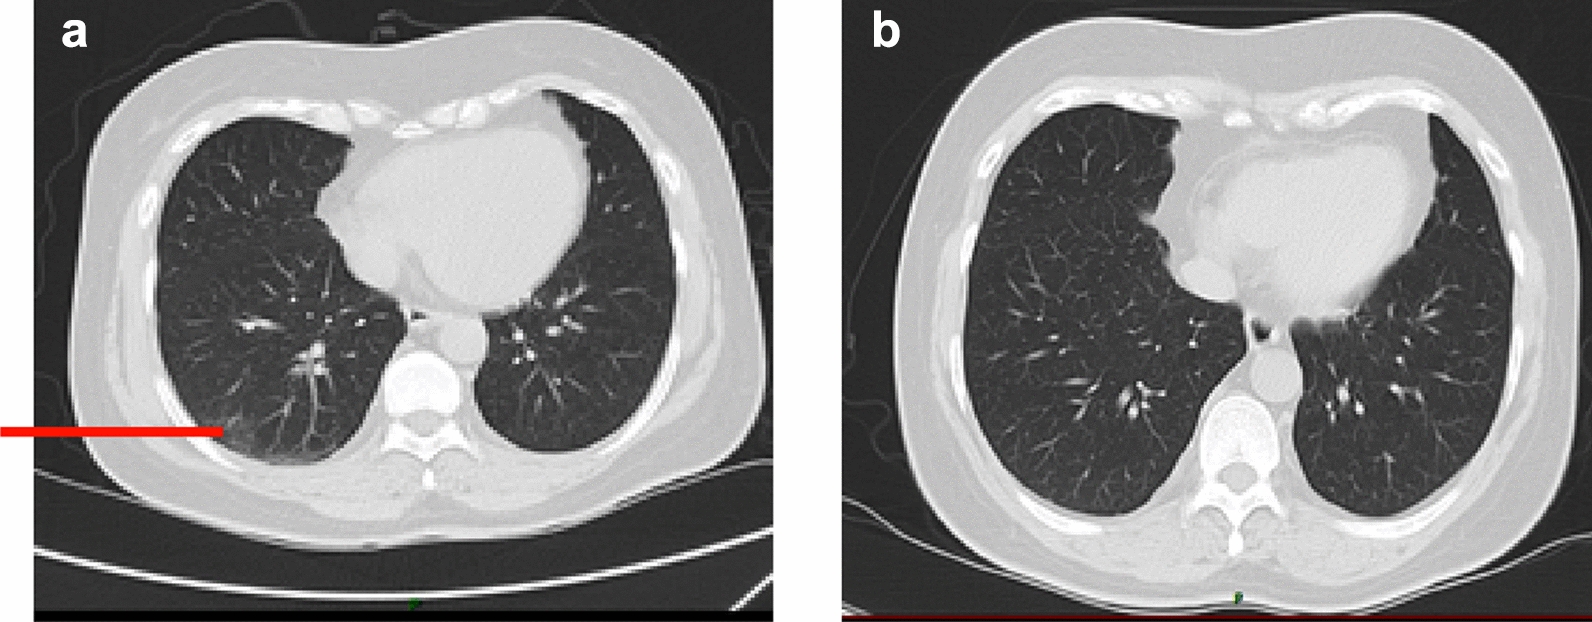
The CT scan on Day 5(a) and Day 15(b)
